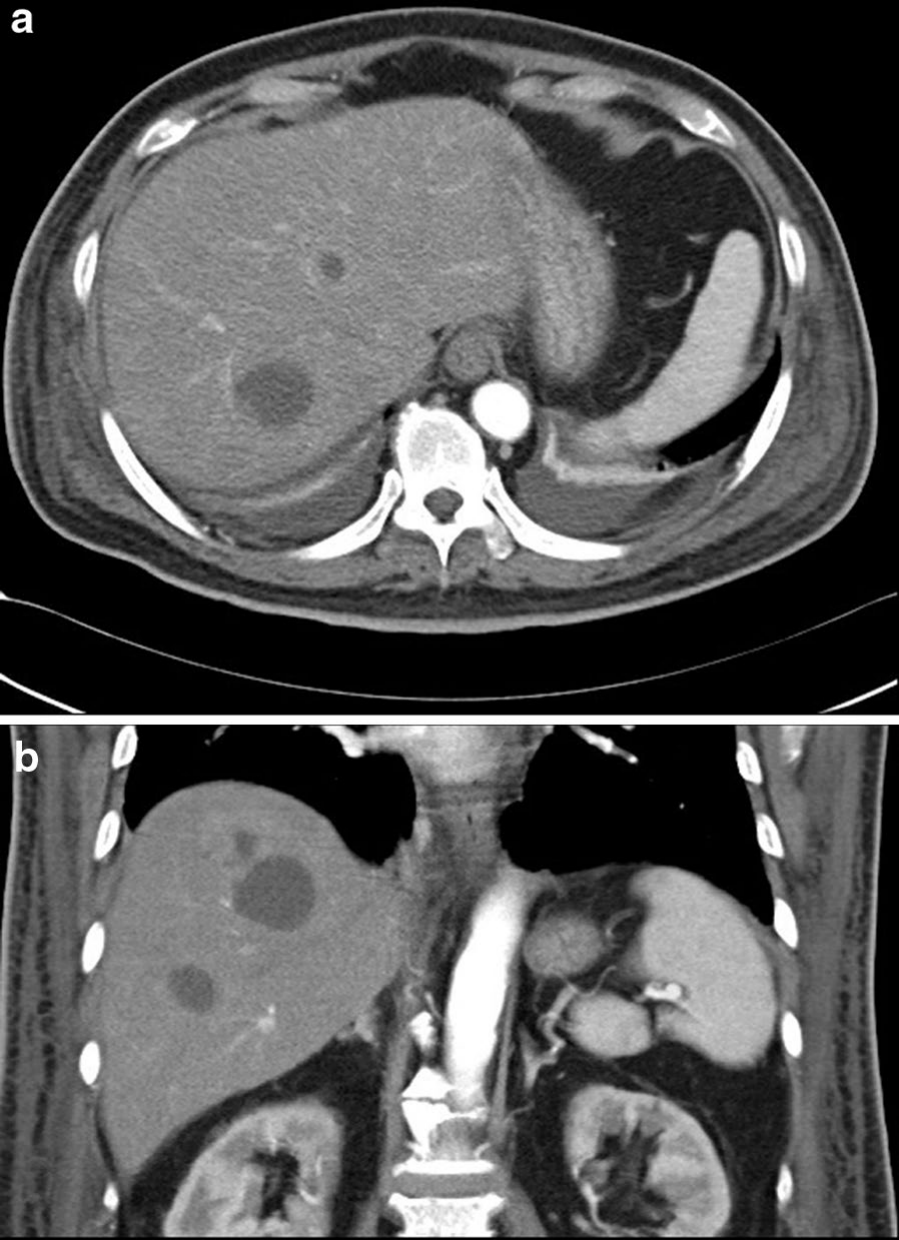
a, b Contrast-enhanced abdominal CT with contrast denoted numerous lobulated hypodensity lesions in liver compatible with liver abscess overlying segment IV, segment V, segment VI, segment VII, and segment VIII
